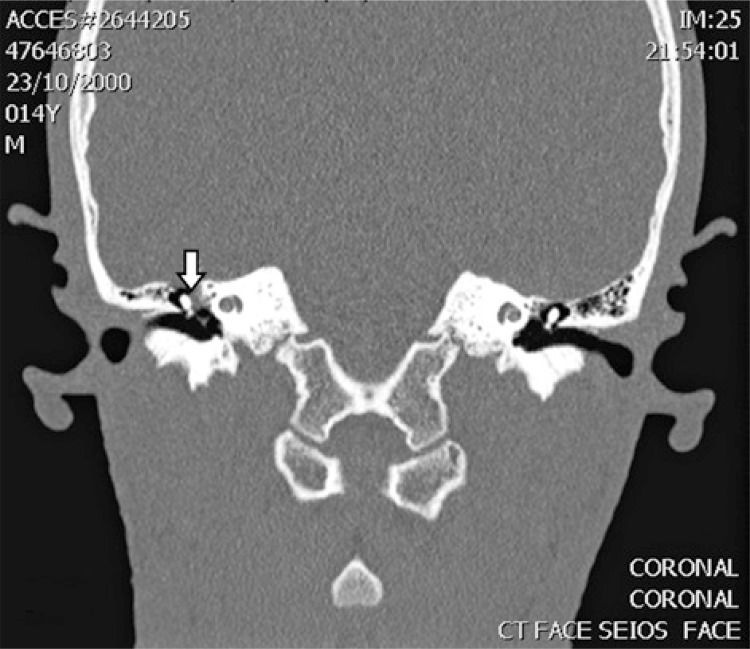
Computed tomography coronal section view of temporal bones showing hemangioma (white arrow) of right facial nerve
